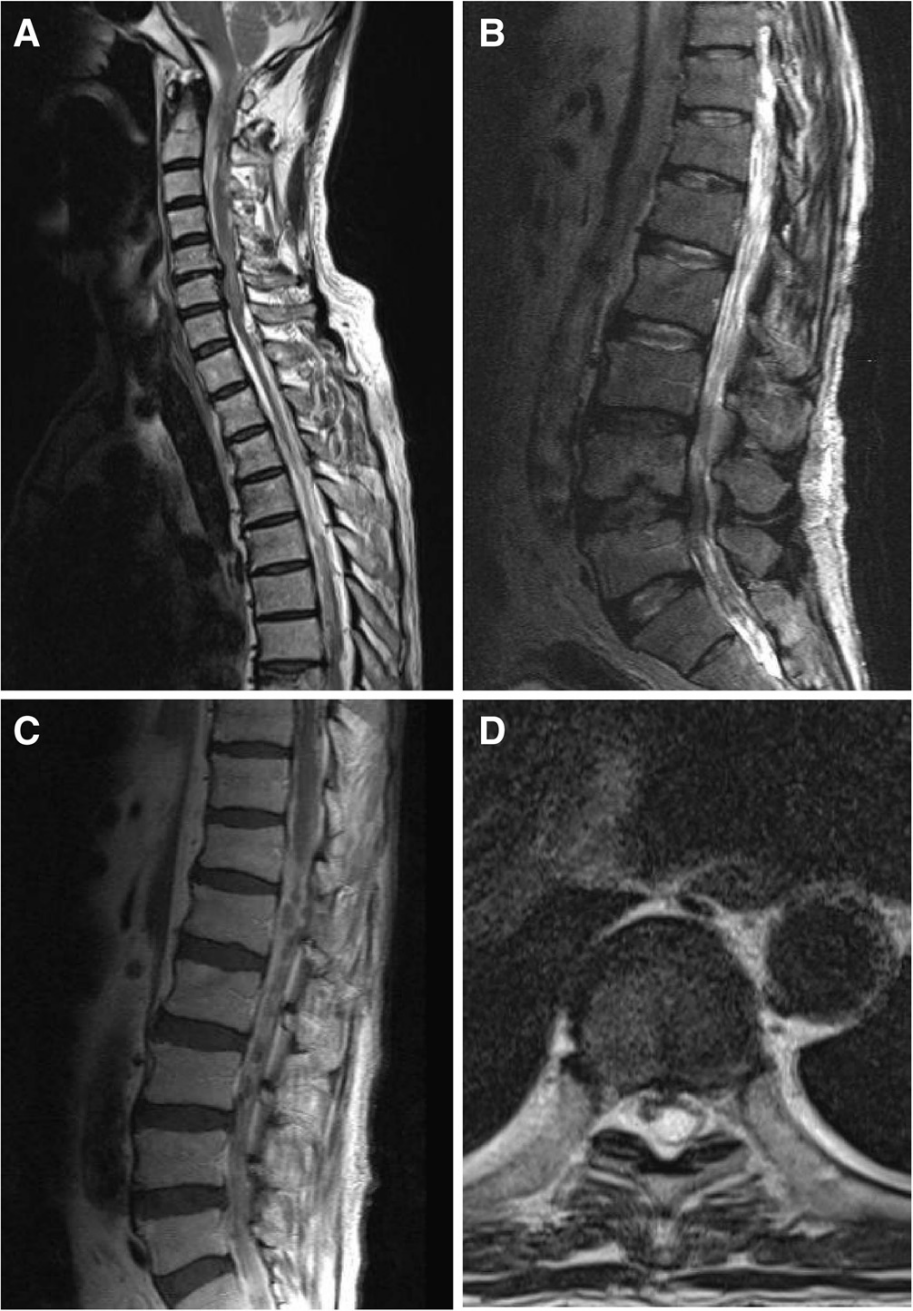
Whole-spine magnetic resonance images of the patient. A, B, C, D: Sagittal and axial T2-weighted images and postcontrast T1-weighted image showing multiple diffuse abnormal signals.
